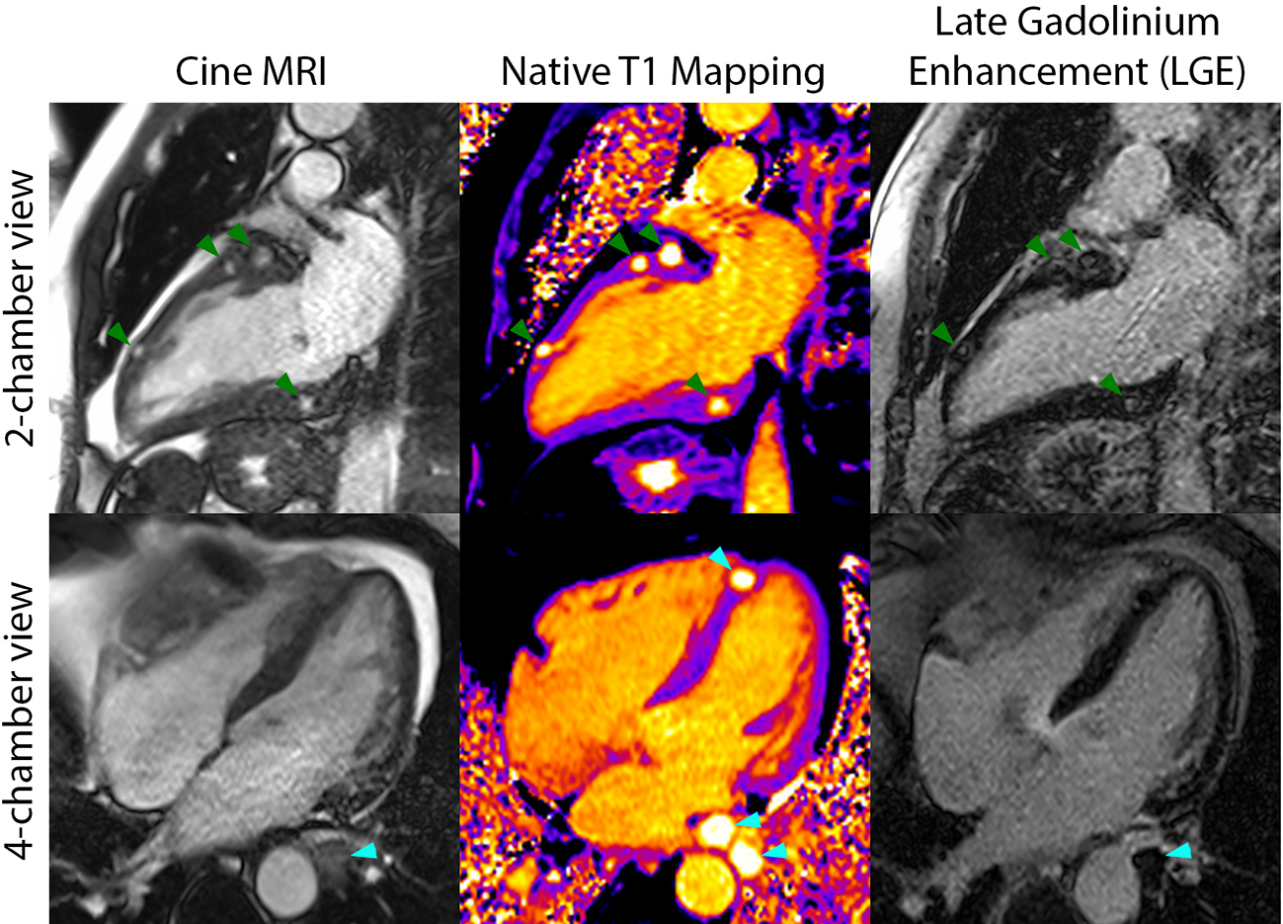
Cardiac magnetic resonance imaging included cine MRI (left column), native T1 mapping (middle column), and late gadolinium enhancement (LGE) imaging (right column) and demonstrated several myocardial metastases on the two-chamber views (green arrows) and three metastases in the four-chamber views (cyan arrows). The metastases were best seen on T1 mapping and cine MRI and had long T1 and T2 (not shown) relative to myocardium.
